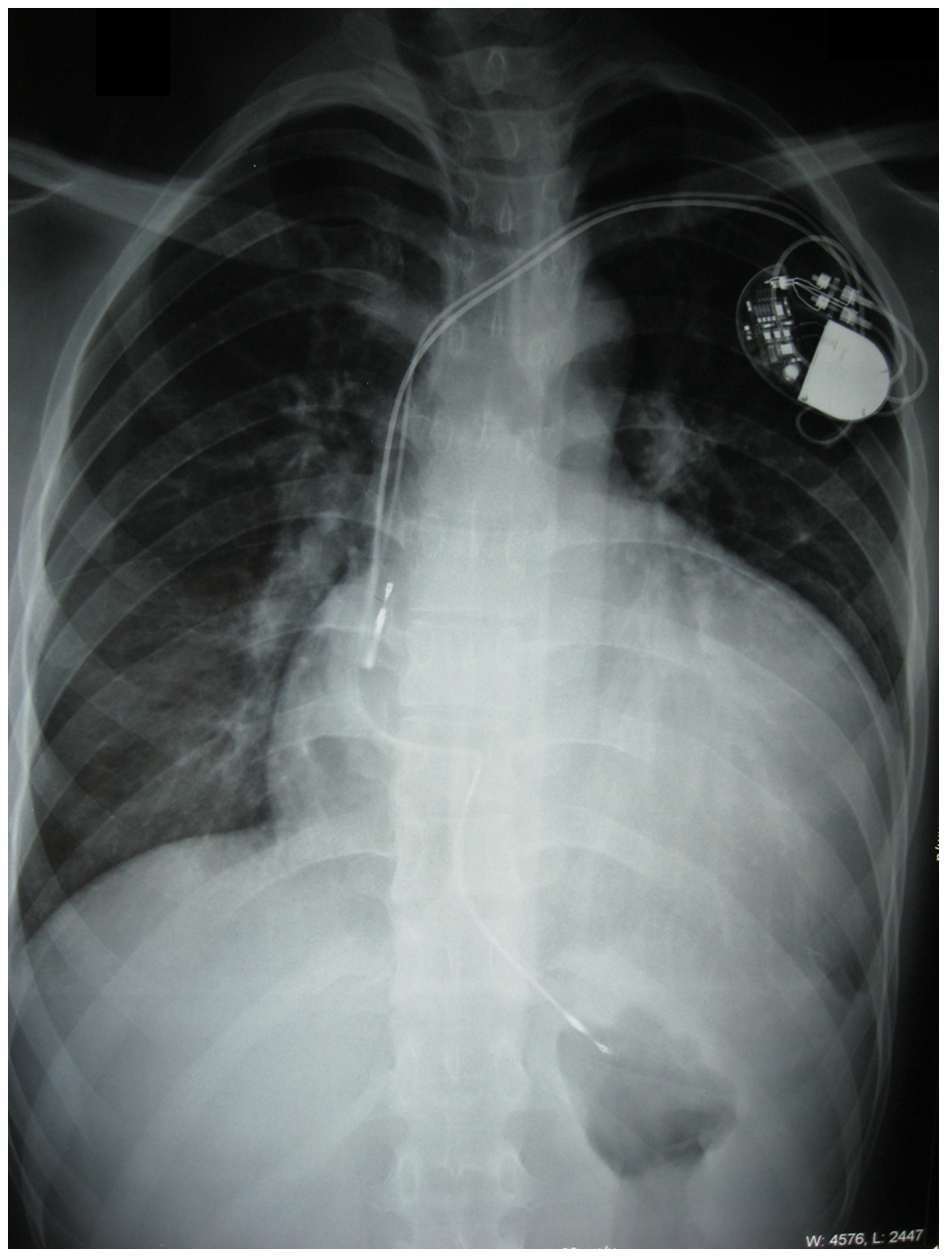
Postero-anterior chest radiograph of the proband after permanent pacemaker implantation. It shows severe cardiomegaly with a cardiothoracic rate of 70.6%.
